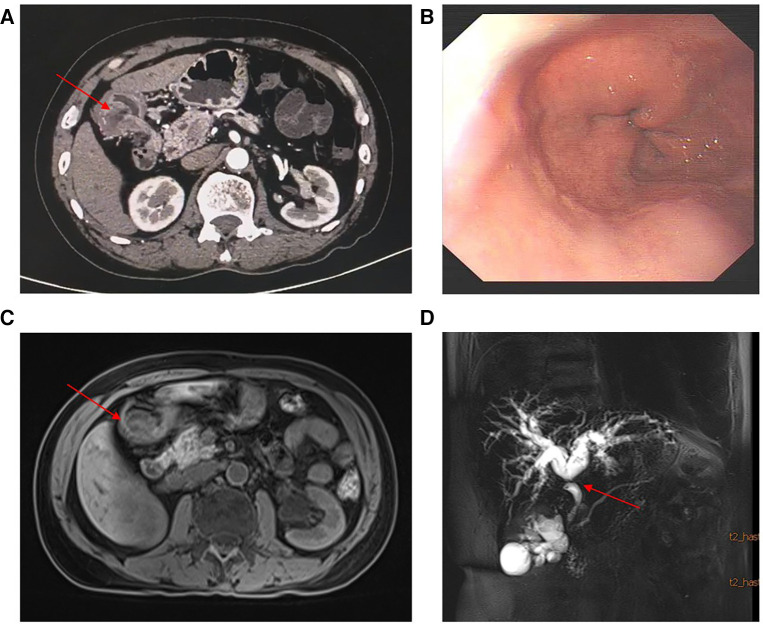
(A) Contrast-enhanced computed tomography showed stenosis in the extrahepatic distal bile duct and a mass with nodular enhancement in the gastric antrum. (B) Gastroscopy showed pyloric stenosis and no mucosal protrusion. (C–D) Enhanced nuclear magnetic resonance and MRCP showed the mass and dilatation of the bile duct and local stenosis of the extrahepatic distal bile duct.
